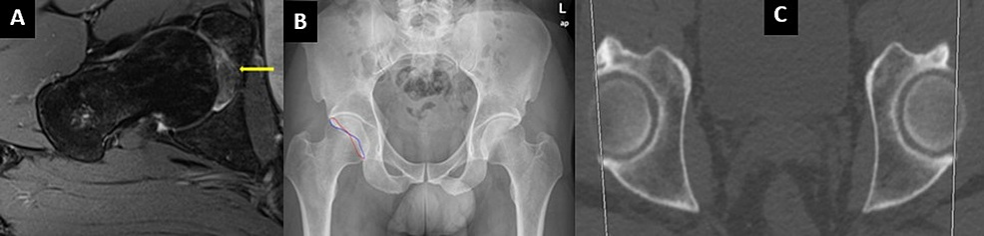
A 45-year-old male who presented with right-sided hip pain. (A) MRI oblique axial PD fat saturation image showing ligamentum teres edema and thickening. (B) X-ray AP pelvis demonstrates bilateral retroversion, more severe on the right side. It shows the figure of 8 appearance (blue: posterior acetabular rim, red: anterior acetabular rim). (C) Axial CT of the hip depicting bilateral retroversion, more severe on the right side. MRI: magnetic resonance imaging; PD: proton density; CT: computed tomography
